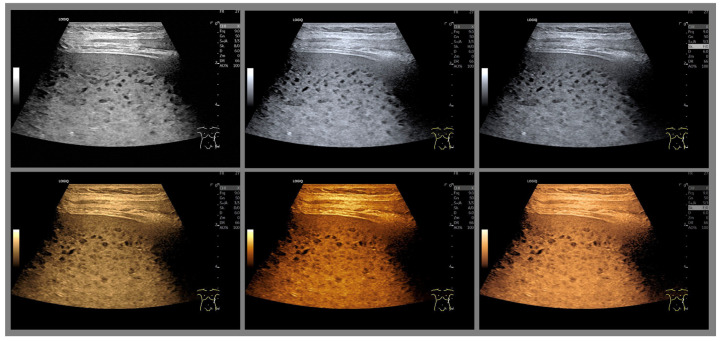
Enlarged spleen with multifocal small hypoechoic parenchymal nodules in an 87-year-old female patient with malignant lymphoma, imaged with 3 different gray map variants and 3 different yellow/brown tones on a GE E10 ultrasound machine (GE HealthCare Technologies, CA, USA).
